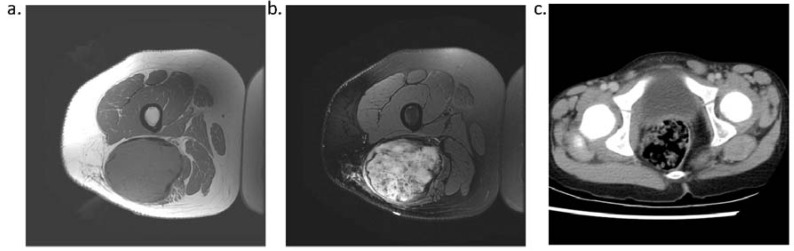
T1 (a) and T2 (b) weighted MRI and contrast-enhanced CT (c) of desmoid tumors provide anatomic information useful in the management of desmoid tumors.
